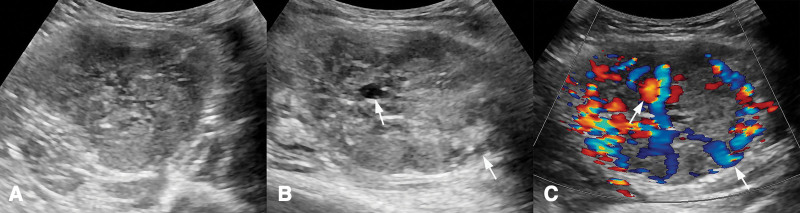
Real-time ultrasound images of the triceps brachii muscle tumor. (A) Sonogram shows a 7.9 × 6.1 × 4.8 cm, well limited, heterogeneous hypoechoic tumor in the triceps brachii muscle. (B) Tube-like echoless is evident in central and peripheral of the tumor (arrows). (C) Color Doppler ultrasound shows abundant blood flow signals in intratumoral and extratumoral areas, and the tubular structures are extremely hypervascularized (arrows).
